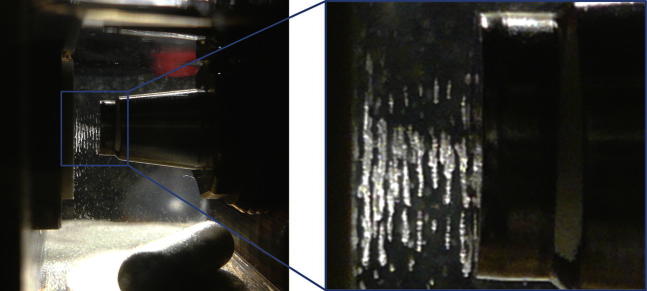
An ultrasonic standing wave is built up between the transducer and the attenuated total reflection (ATR) probe acting as reflector. The enlargement on the right-hand side shows the half-wavelength structure of the particle agglomerates (polystyrene beads were used here for demonstration purposes). By changing the frequency, the location of the pressure nodal planes is altered, and therefore, the evanescent field of the ATR is either populated with particles or depopulated. Taken from: Brandstetter M. Ultrasonically enhanced in-line attenuated total reflection (ATR) infrared absorption spectroscopy of suspensions. Master Thesis, Vienna University of Technology, 2009.
